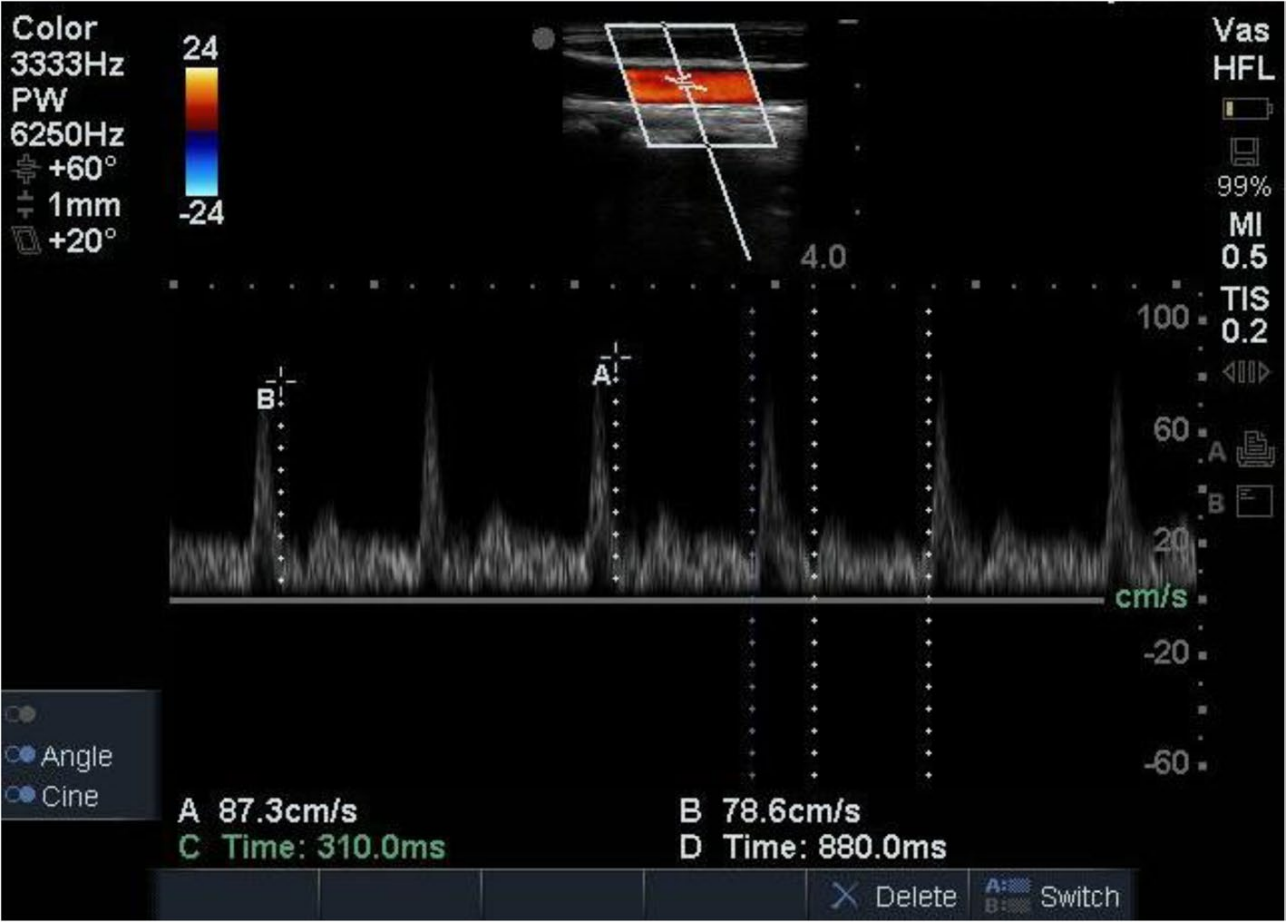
Carotid FTc and ΔVpeak measurement with Doppler ultrasound.Time C is FT.*FTc* carotid corrected flow time,*ΔVpeak* respirophasic variation in carotid artery blood flow peak velocity,*FT* flow time
